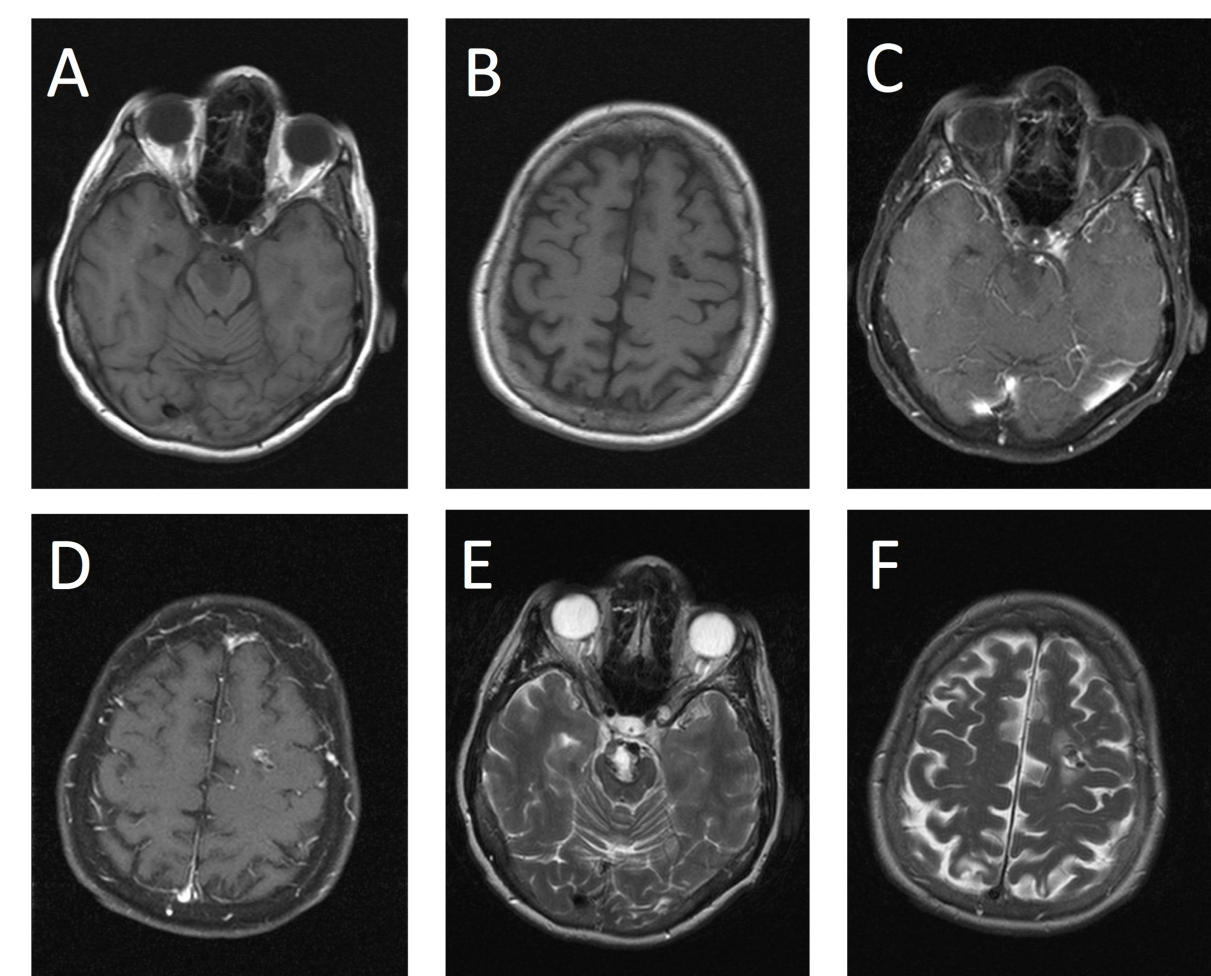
MRI demonstrating multiple CAPNONs Magnetic resonance imaging (MRI). T1-weighted images pre-contrast depicting two hypointense masses located in the ventral brainstem (A) and left frontal lobe (B). T1-weighted images post-contrast showing no contrast enhancement in the brainstem lesion (C) and heterogeneous enhancement on the left frontal lesion (D). T2-weighted images demonstrate T2 hyperintensity (E) and edema around the left frontal mass (F).
